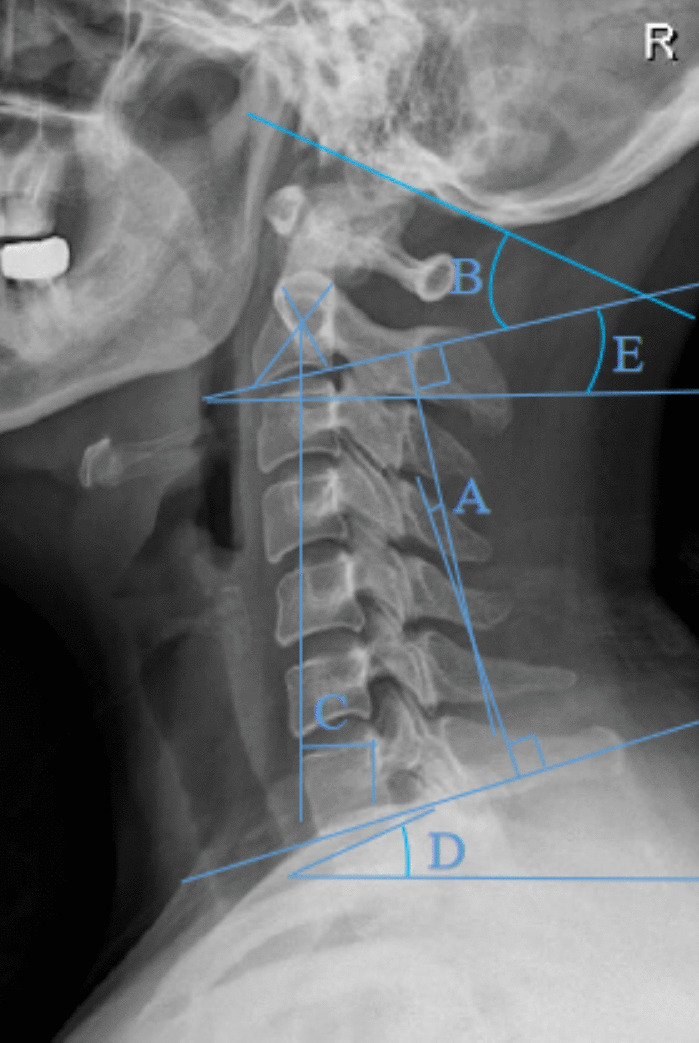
Radiographic measurements. A C2‒C7 Cobb angle. B C0‒C2 Cobb angle. C C2–C7 Sagittal Vertical Axis (SVA). D T1 slope. E C2 slope
